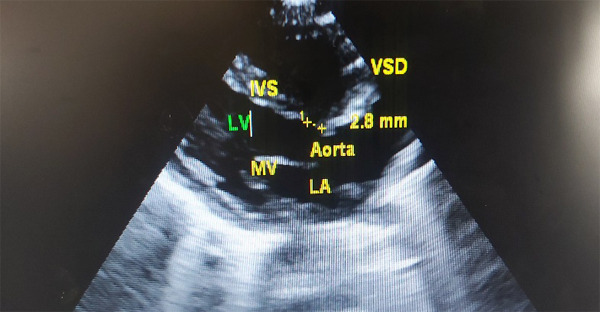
2D echocardiography showing a 2.8 mm Ventricular Septal Defect.
